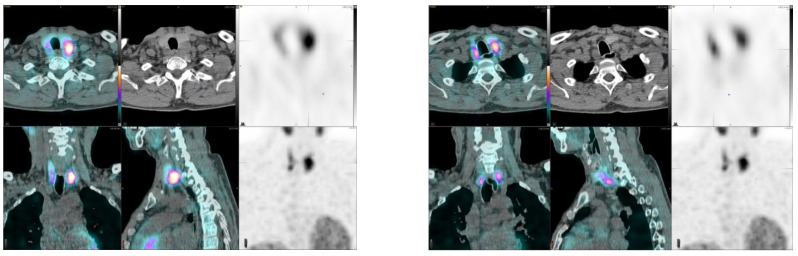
A 55-year-old male with hyperparathyroidism, right inferior adenoma, left parathyroid carcinoma. His S-PTH concentration was 478 ng/L and S-Ca 11.1 µg/L. The SUVmax values of the right-sided adenoma were 6.3 (CGZAS) and 3.8 (CGAS), respectively. Visually, this uptake was weak (1 < TBR < 2) and lesion diameters on CT were 21 mm × 24 mm. The carcinoma on the left demonstrated higher SUVmax values than the adenoma: 8.3 (CGZAS) and 7.3 (CGAS), respectively. Visually this uptake was moderate (2 < TBR < 3) and the lesion diameters on CT were 25 mm × 30 mm. This patient was not operated because of MEN syndrome.
